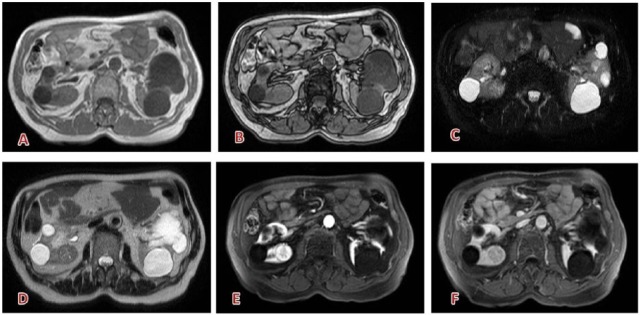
Typical clear-cell carcinoma of the right kidney: well-defined capsulated solid lesion, (A) hypointense on T1-weighted, with subtle signal loss in the out-of-phase sequence (intracellular fat, B); (C) partially restricted diffusion, inhomogeneous; (D) slightly hyperintense on T2; (E) strong and rapid enhancement in corticomedullary phase; (F) best seen on nephrogenic phase. Contrast agent: gadobutrol, 6.5 mL. Courtesy of Professor De Cobelli, Radiology Department, San Raffaele Scientific Institute, Milan.
